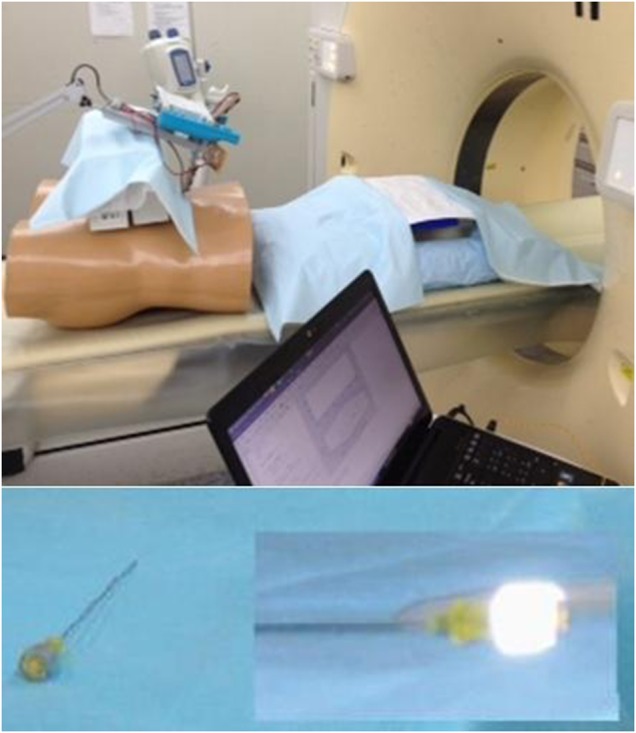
Experimental setup. The CT scanner is on the right, and the IR cameras to the left
